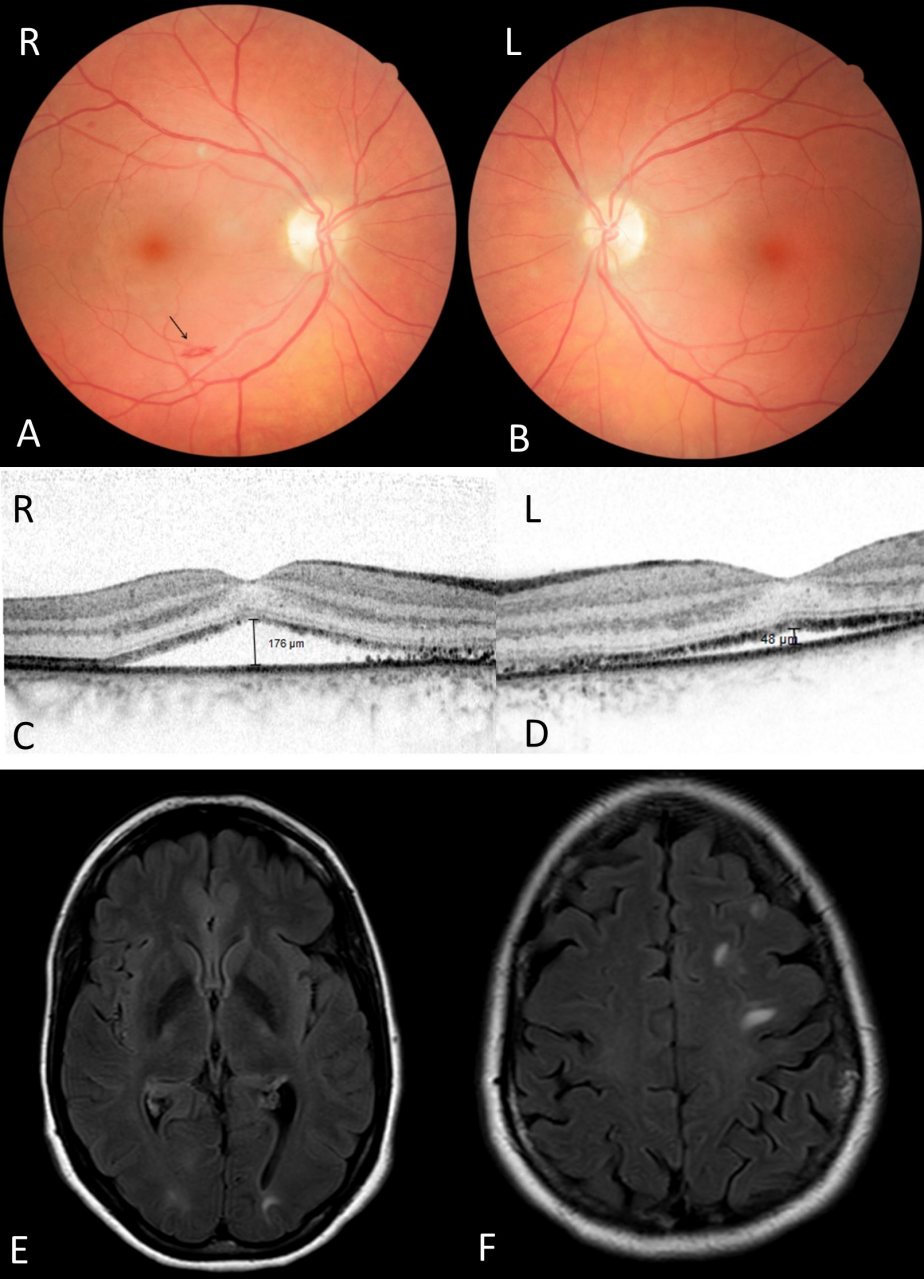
Retinal and radiological changes in PRES. A, B: Funduscopic image of patient 2 with PRES at baseline showing a small bleeding (arrow) in the right eye. C, D: OCT of patient 2 with PRES at baseline showing bilateral subretinal fluid. E: MRI image of patient 2 with PRES showing hyperintensity in the temporo-parietal region. F: MRI image showing hyperintensity in the occipital region.
